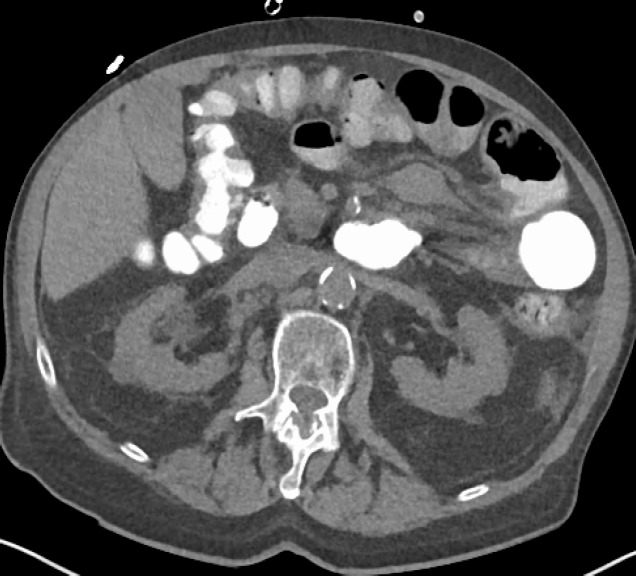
Small mesenteric mass (yellow arrow) which was FDG positive on the previous PET scan
